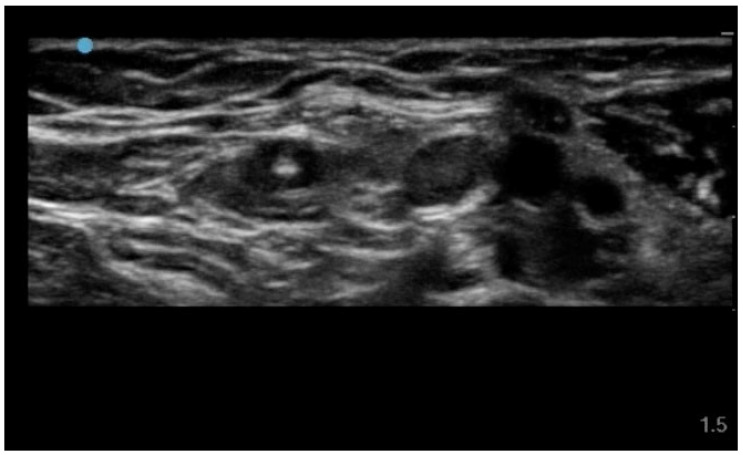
Ultrasound visualization of central intraluminal needle tip position.
